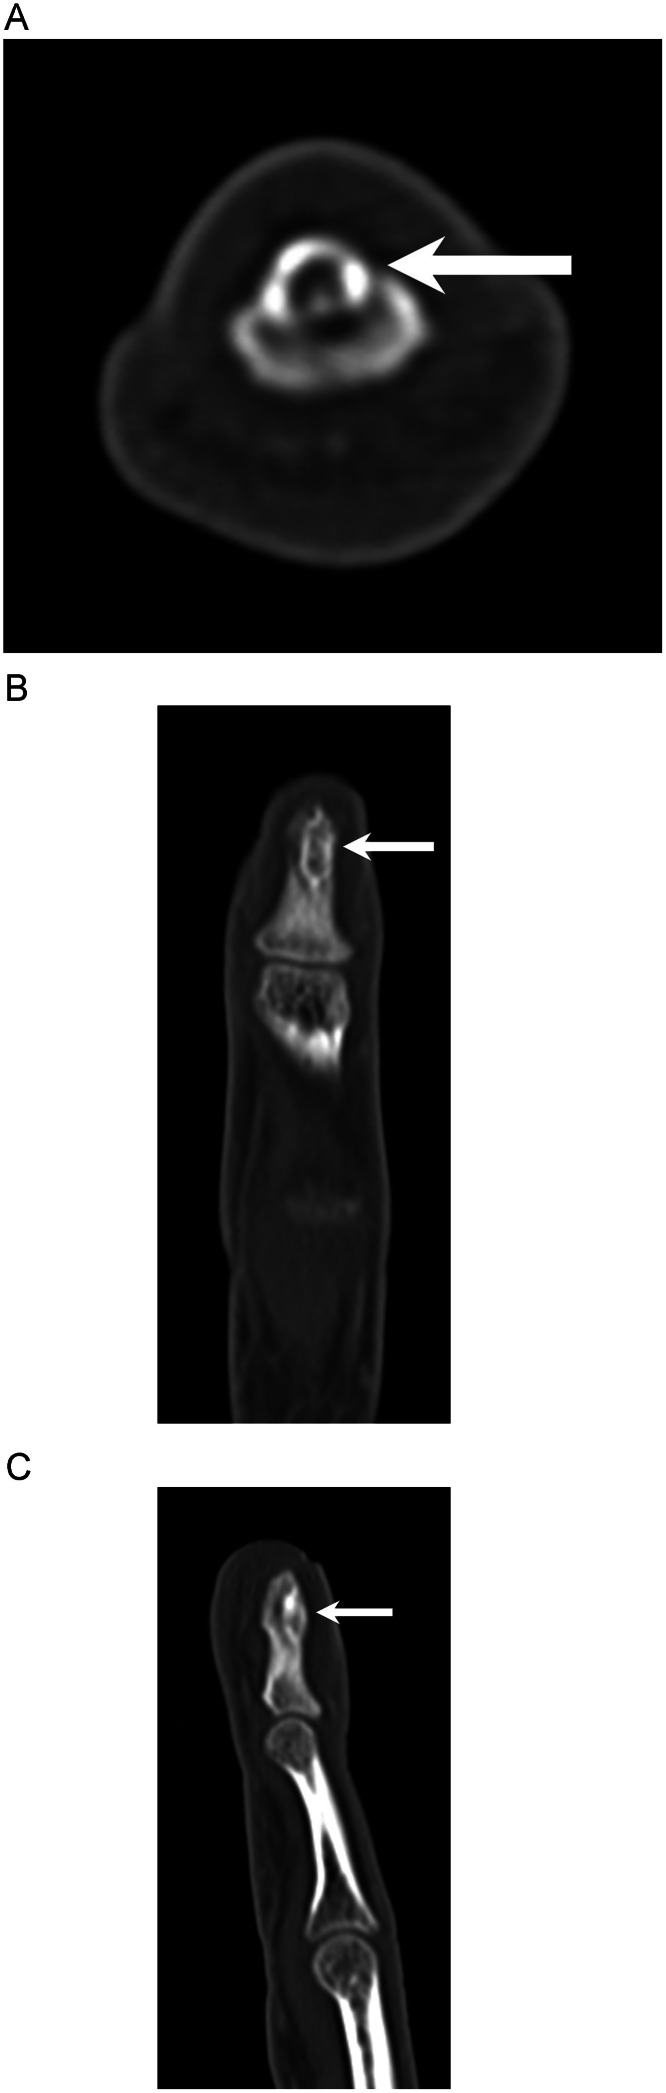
(A, B, C) Axial, coronal, and sagittal CT images of the left middle finger show an ovoid lucent lesion with sclerotic margins in the left middle finger distal phalanx tuft. The lesion measures approximately 6 mm long by 2 mm deep by 3 mm transverse. The lesion appears expansile and is centered in the dorsal cortex with dorsal bony protuberance.
